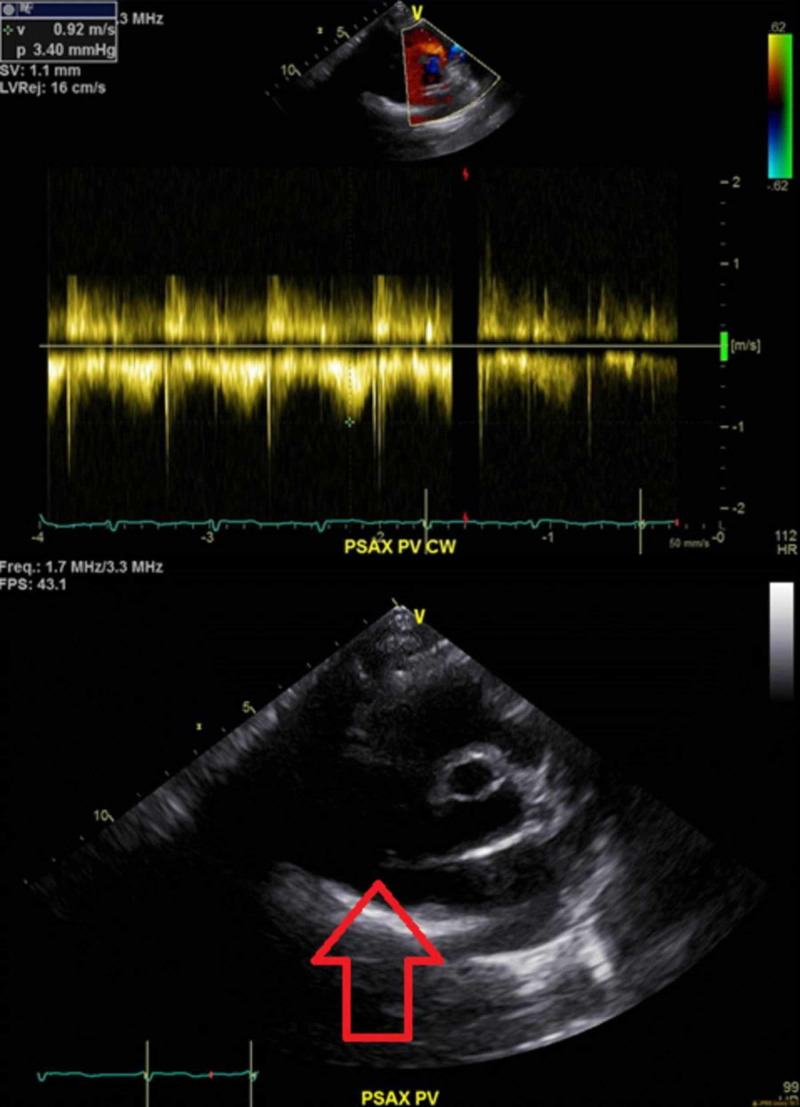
TTE. No evidence of vegetations on the valves. TTE, transthoracic echocardiogram
